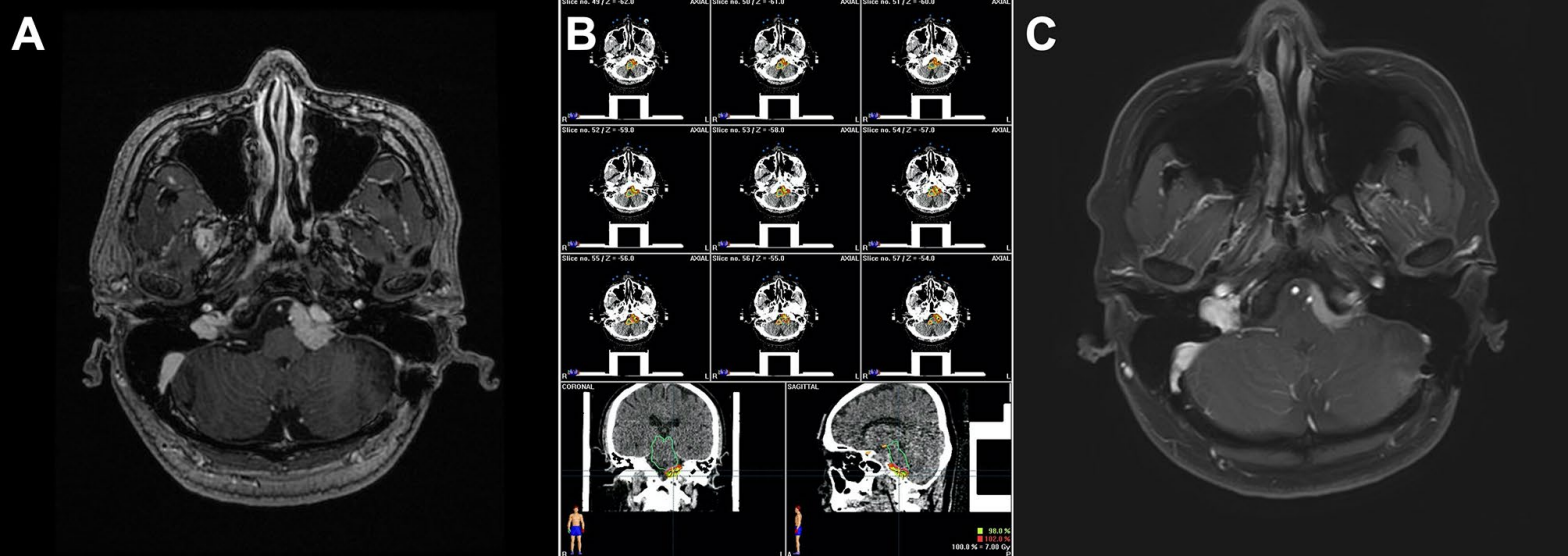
A 48-year-old male with a petroclival meningioma (volume, 3.02 ml). Treatment was 21 Gy in three fractions. (A) Pre-treatment magnetic resonance imaging (MRI) scan. (B) Treatment plan showing the resulting isodose line. (B) MRI scan 36-months after treatment showing tumor regression.
